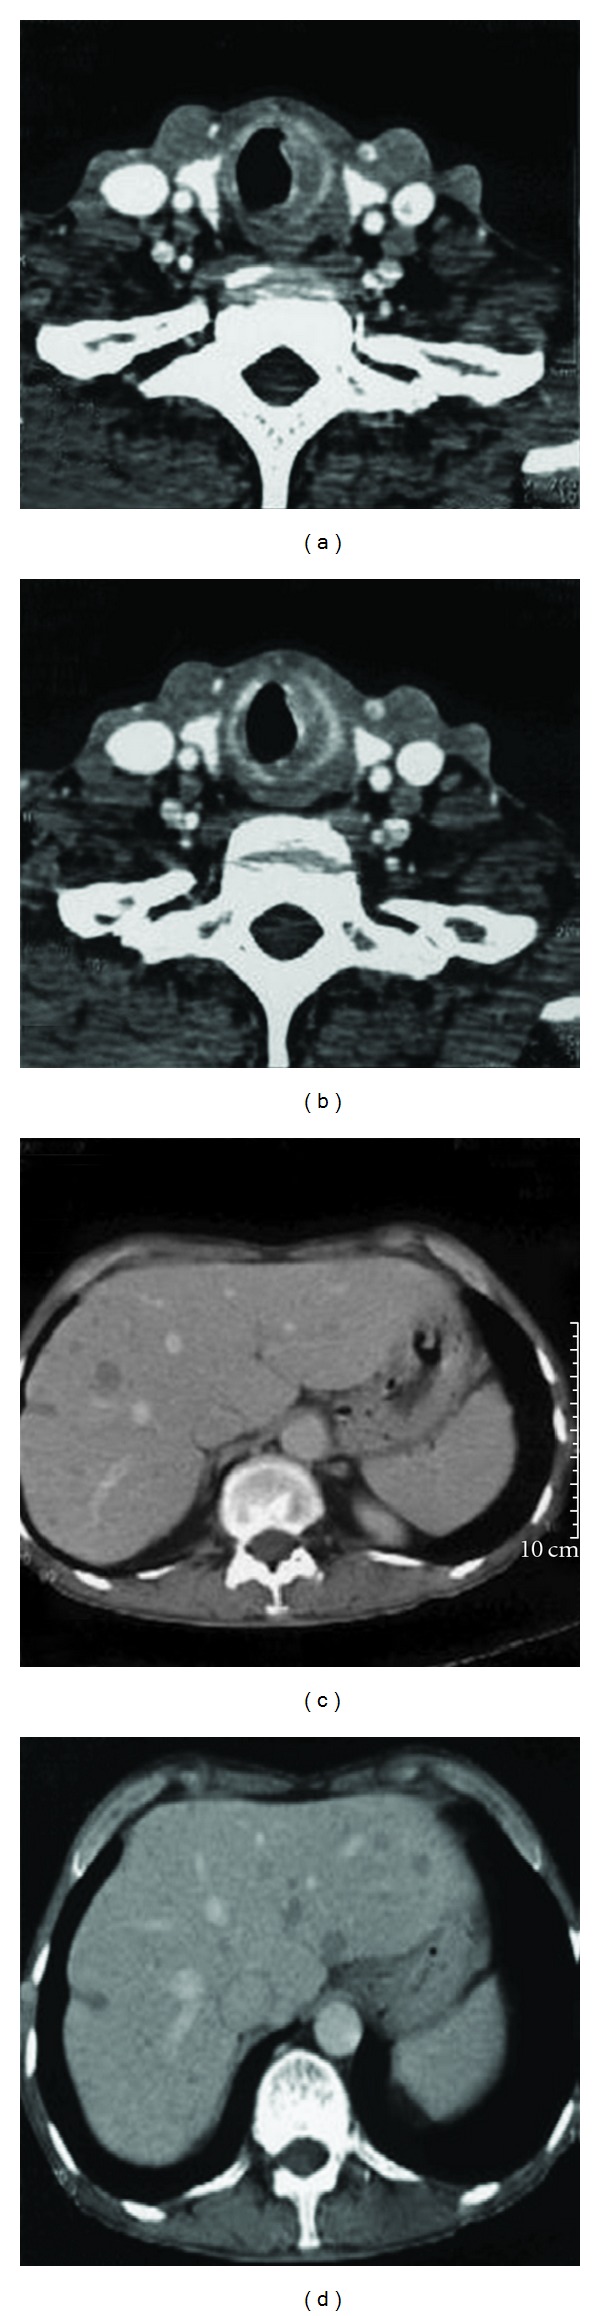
CT scan of the patient showing the primary tumor in the subglottic region.
